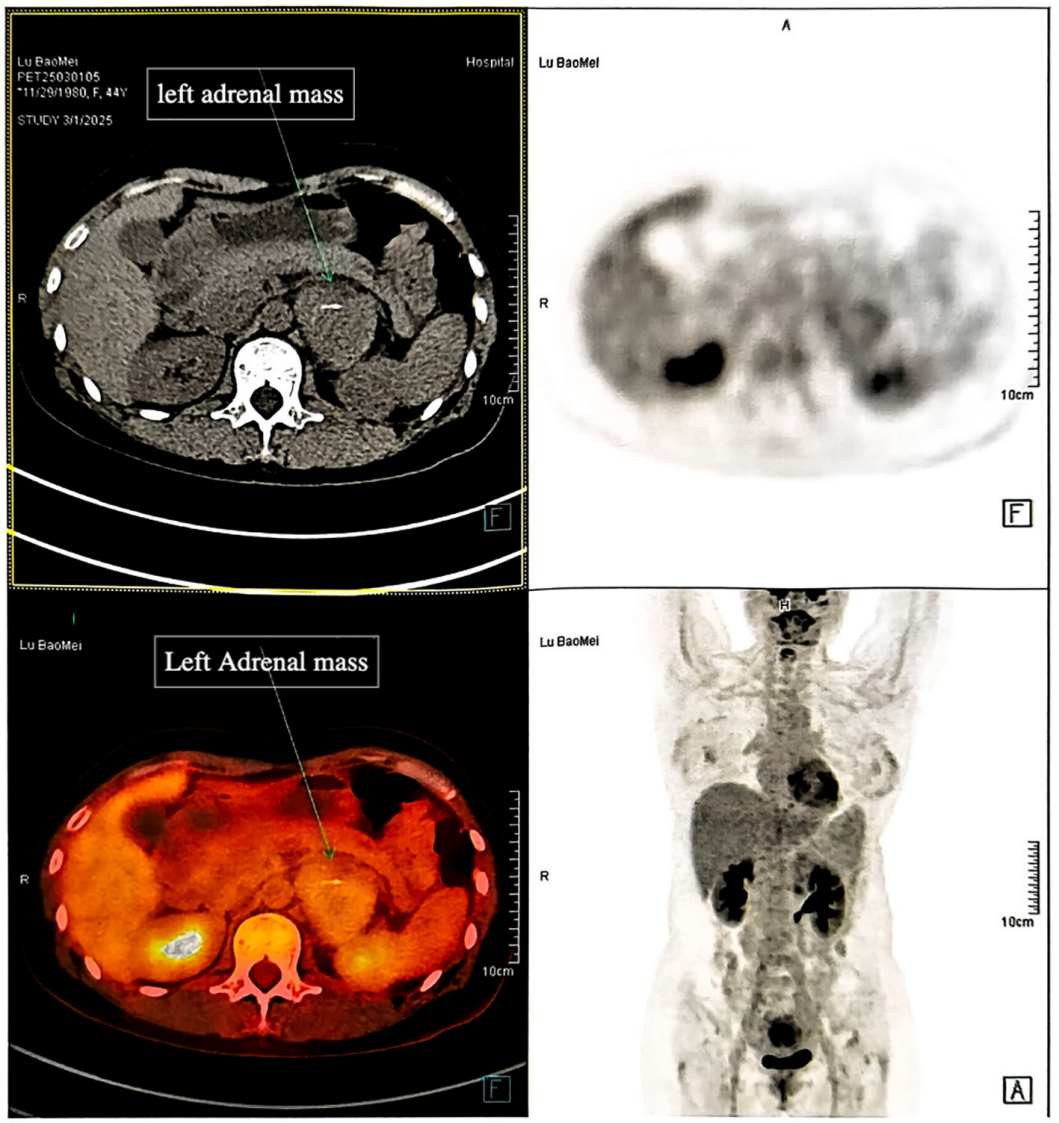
PET-CT scan demonstrating increased FDG uptake in the left adrenal region lesion.
